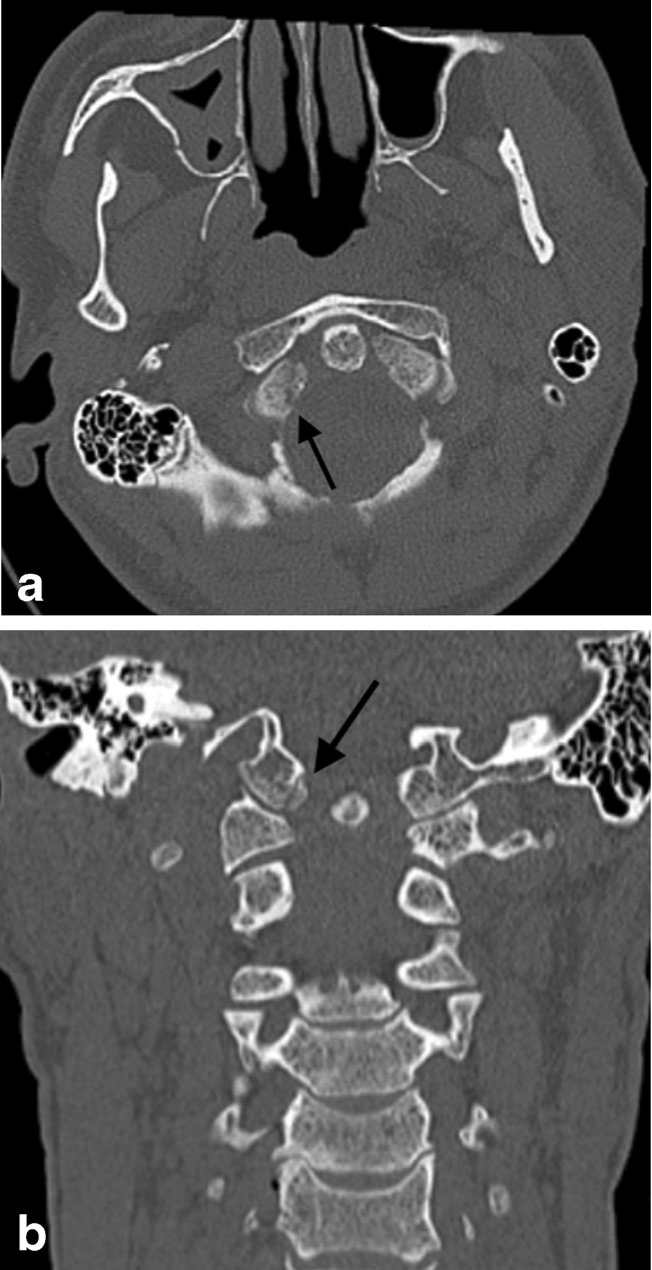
Type III occipital condyle fracture: a axial CT image through the atlanto-occipital level performed as part of a polytrauma CT assessment of a young female pedestrian struck by a motorcyclist travelling at speed; b coronal CT image of the same patient. The type III occipital condyle fracture can be subtle on CT imaging [indicated here on the right (*arrow*)] but may indicate a high-energy traumatic mechanism to the craniocervical junction with potentially unstable craniocervical spine injury related to alar ligament avulsion
